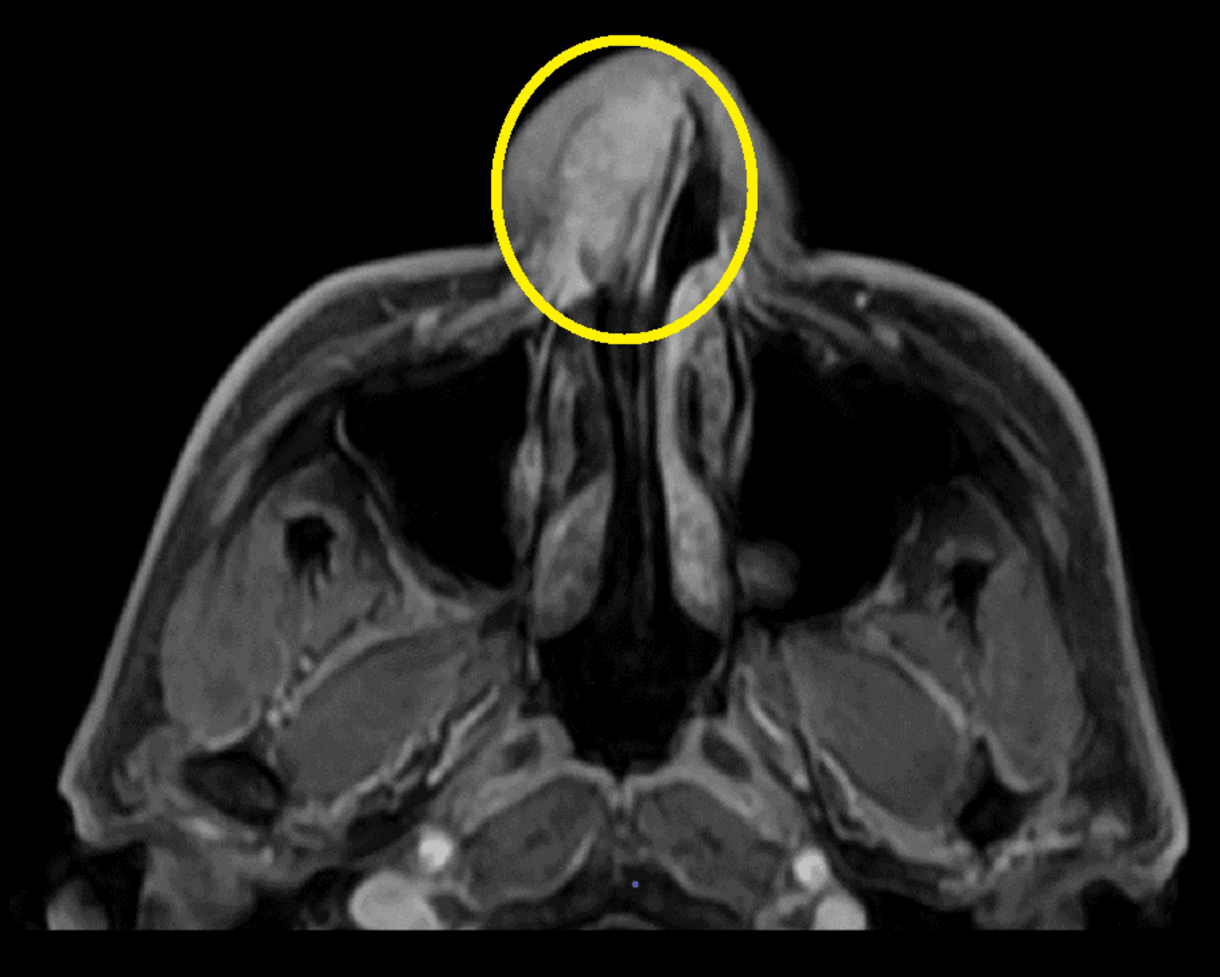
magnetic resonance imaging. The area enclosed by the circle is the tumor.
